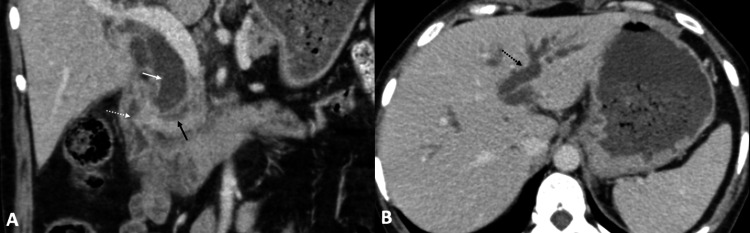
Ampullary involvement Coronal (Figure 3A) and axial (Figure 3B) sections of the CECT of the abdomen show a periampullary mass (dashed white arrow) with dilation of the CBD (white arrow) and pancreatic duct (black arrow). The intrahepatic biliary radicals are dilated as well (dashed black arrow in Figure 3B). CECT: contrast-enhanced computed tomography, CBD: common bile duct
